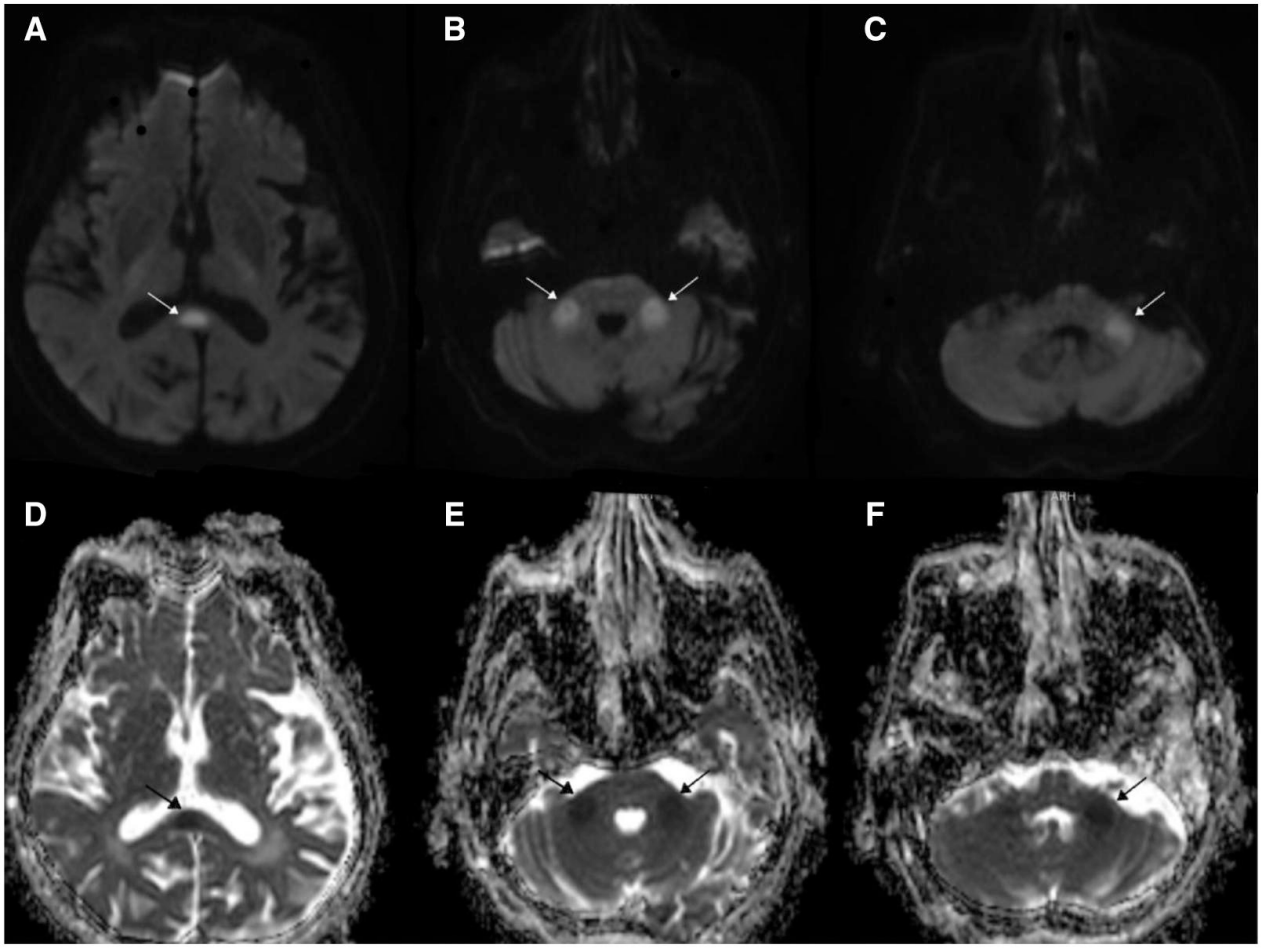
In diffusion-weighted MRI, white arrows indicate restricted diffusion: (a) shows involvement of the splenium of the corpus callosum, while (b) and (c) demonstrate similar findings in the right and left middle cerebellar peduncles (MCP), respectively. The corresponding ADC maps show hypointensity at the same locations, marked by black arrows: (d) in the splenium, and (e) and (f) in the right and left MCPs, confirming cytotoxic edema.
